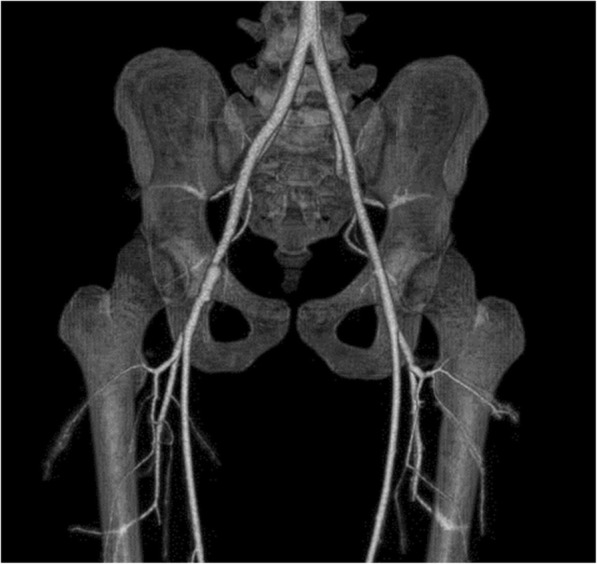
Postoperative CT angiography shows a patent right EIA and CFA and a successful repair. CT, computed tomography; EIA, external iliac artery; CFA, common femoral artery
